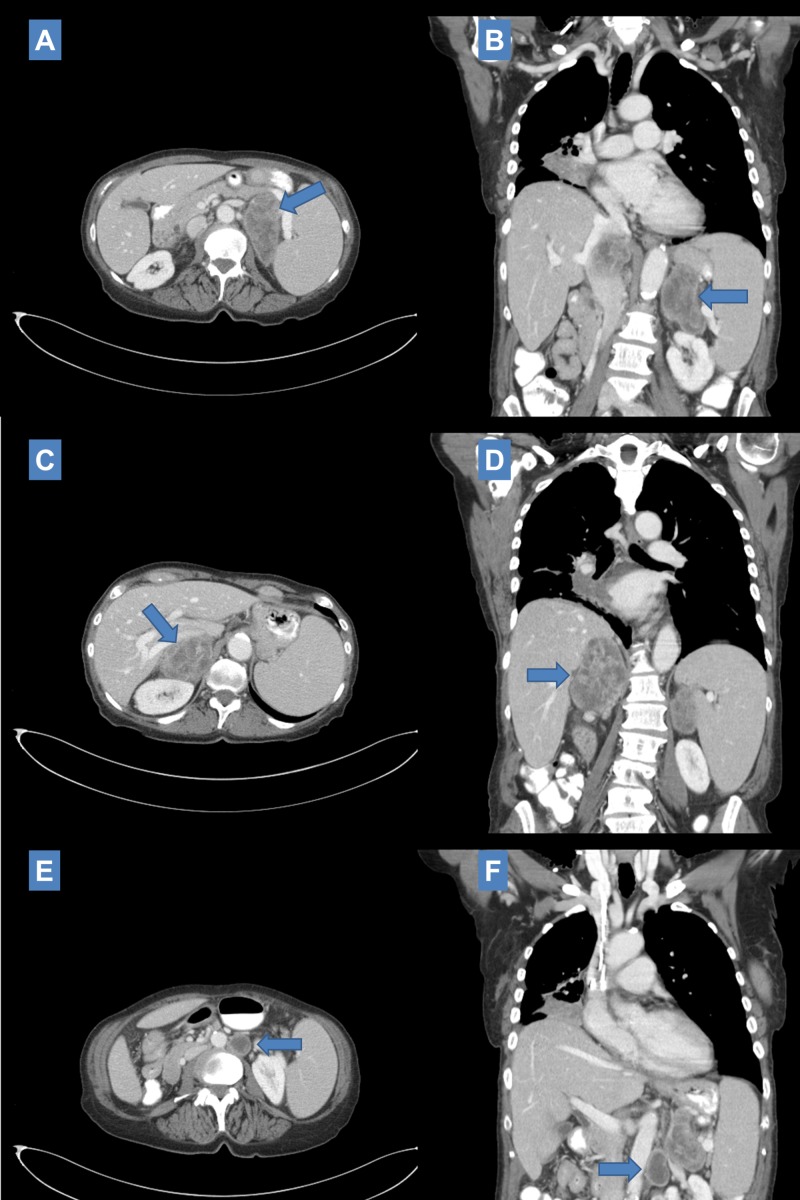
Five-month post definitive treatment computed tomography (CT) scan showing continued enlargement of left adrenal metastasis (8.0 cm x 3.9 cm
